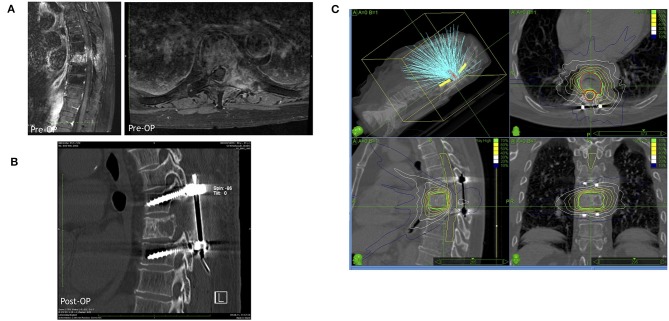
A representative case for a hybrid therapy with a prior surgery including decompression and stabilization followed by a radiosurgical treatment by Cyberknife. (A) Representative MRI images (left in sagittal view and right in axial view) of the metastatic lesion in thoracic vertebra 8. (B) Postoperative CT scan demonstrating the decompression in the lesional segment and dorsal spinal fusion of thoracic vertebra 7 and 9. (C) The Cyberknife treatment planning of the lesional vertebra body after surgery.
